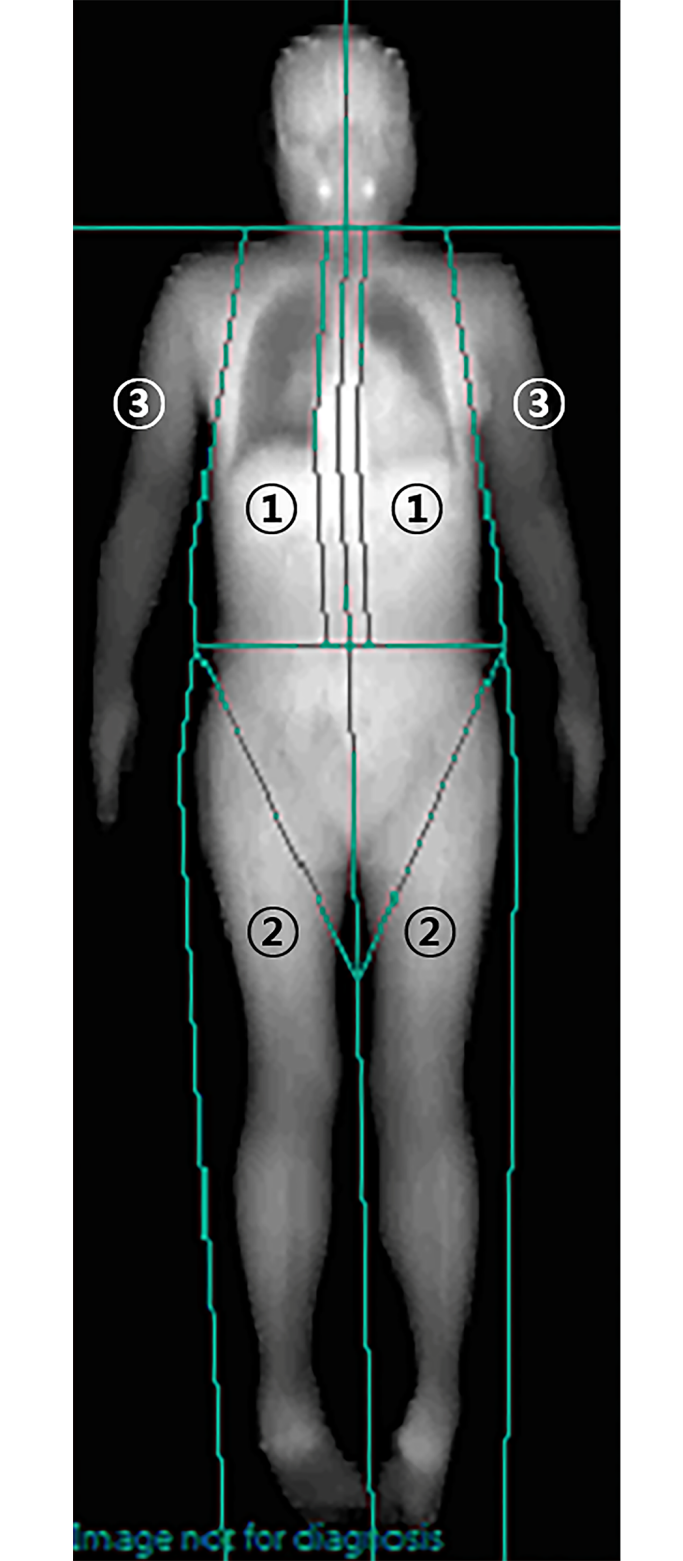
Regional fat measurement by dual-energy X-ray absorptiometry. 1: Trunk, the area bordered by the chin, the iliac crests, and the lateral borders of the ribs; 2: Legs, from the hip joints to the feet; 3: Arms, from lateral borders of the ribs to the arms.
